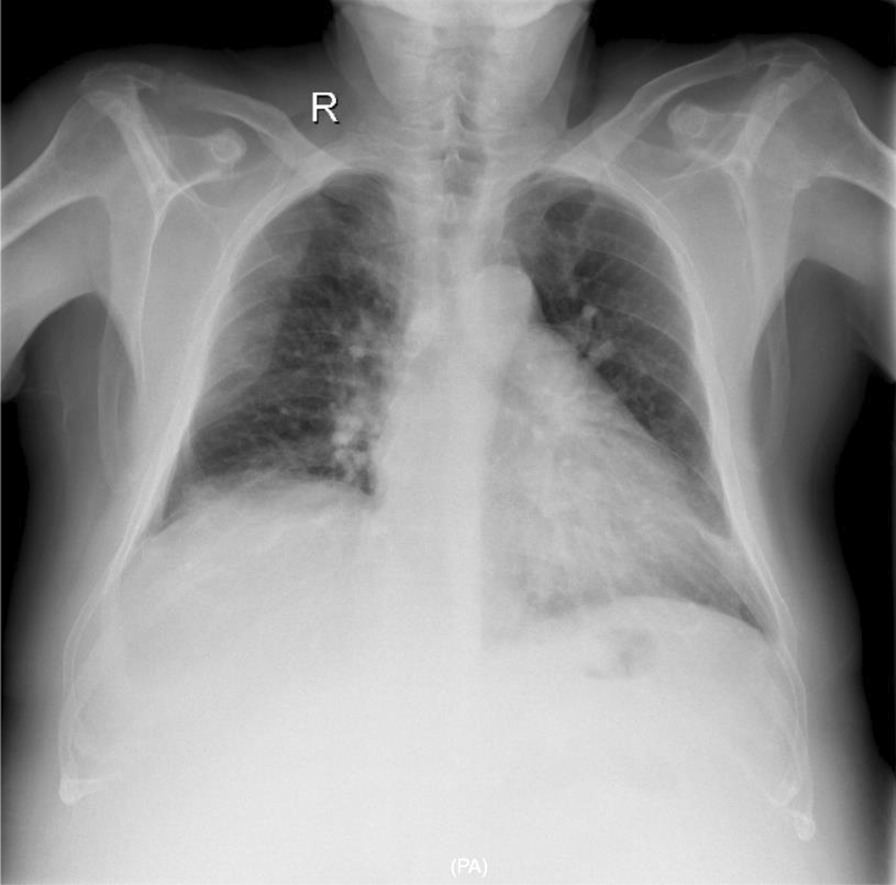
Patient’s chest X-ray on admission
